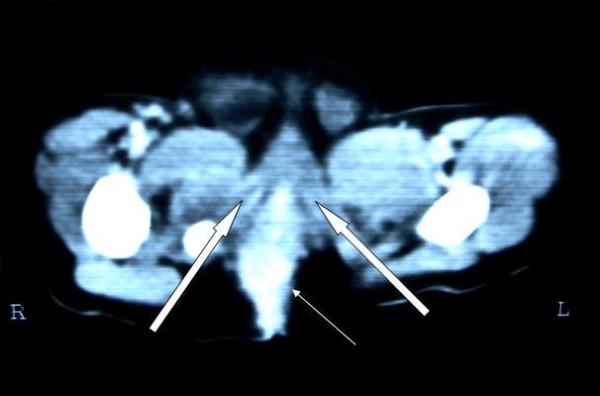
Follow up CT scan showing rectum (straight arrow) placed in midline to the muscle complex (block arrows).
